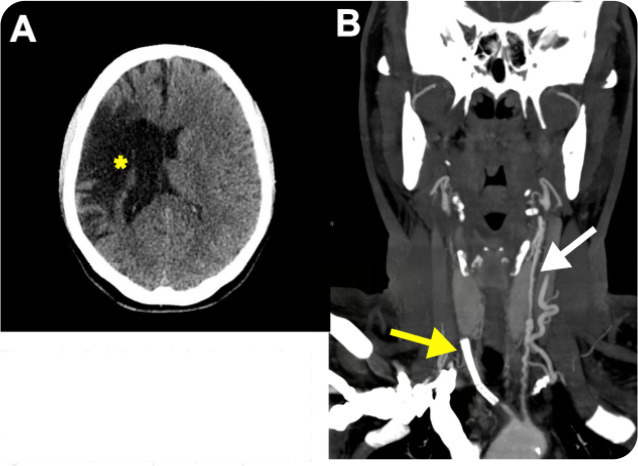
Radiological findings in Case 1 from the first family (the 42-year-old sister). (A) Axial view of a non-contrast brain CT showing a large area of encephalomalacia (yellow asterisk) in the right cerebral hemisphere, a sequela of a chronic ischemic stroke. (B) Coronal view of a CT angiogram of the cervical arteries showing an occluded stent in the right common carotid artery (yellow arrow) and severe stenosis of the left common carotid artery (white arrow).
